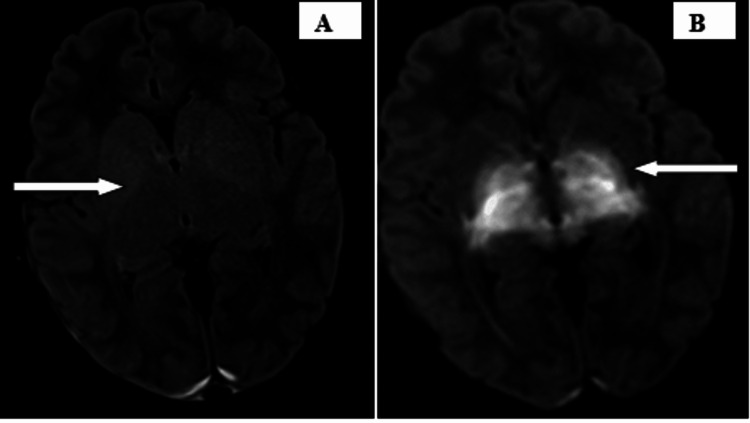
Subtle FLAIR hyperintense signals are noted in the bilateral globus pallidum, thalamus, genu, and the posterior limb of the internal capsule (A). Diffusion restriction is noted in the bilateral globus pallidum, thalamus, genu, and posterior limb of the internal capsule (B). FLAIR: fluid-attenuated inversion recovery
